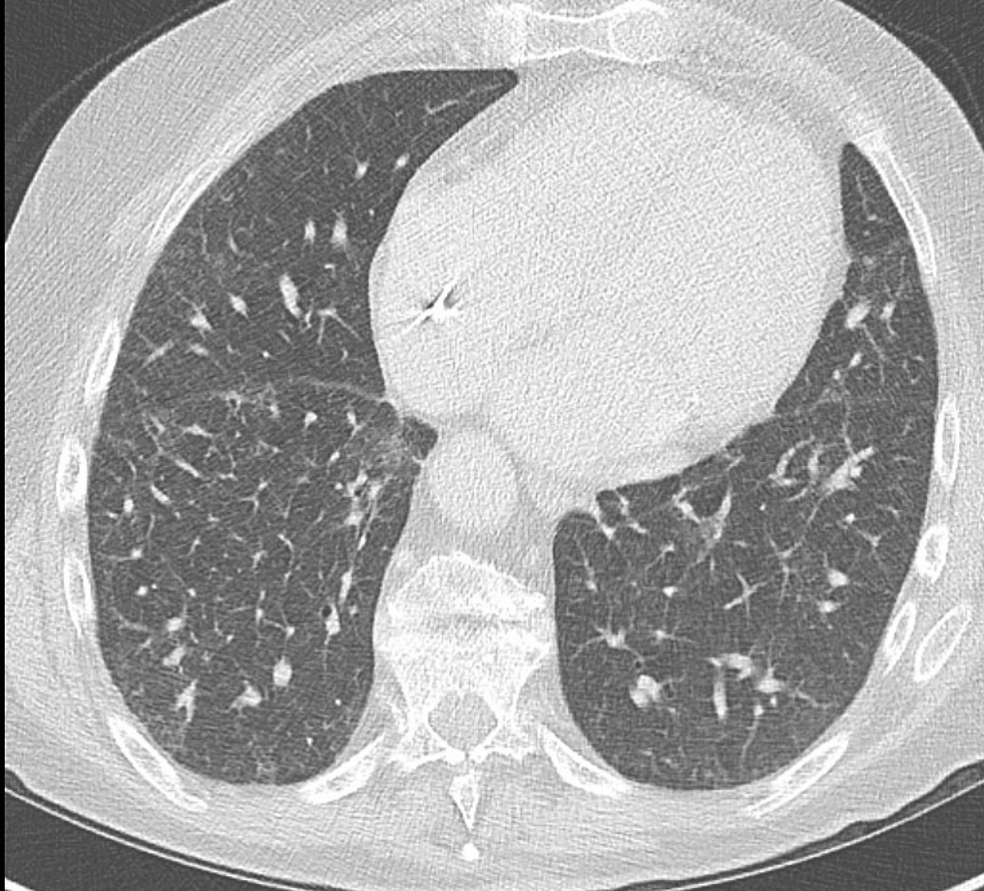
The patient's high-resolution chest CT three months after initiation of mepolizumab showing significant interval improvement in bilateral ground-glass opacities in the mid-lower lung zones.
